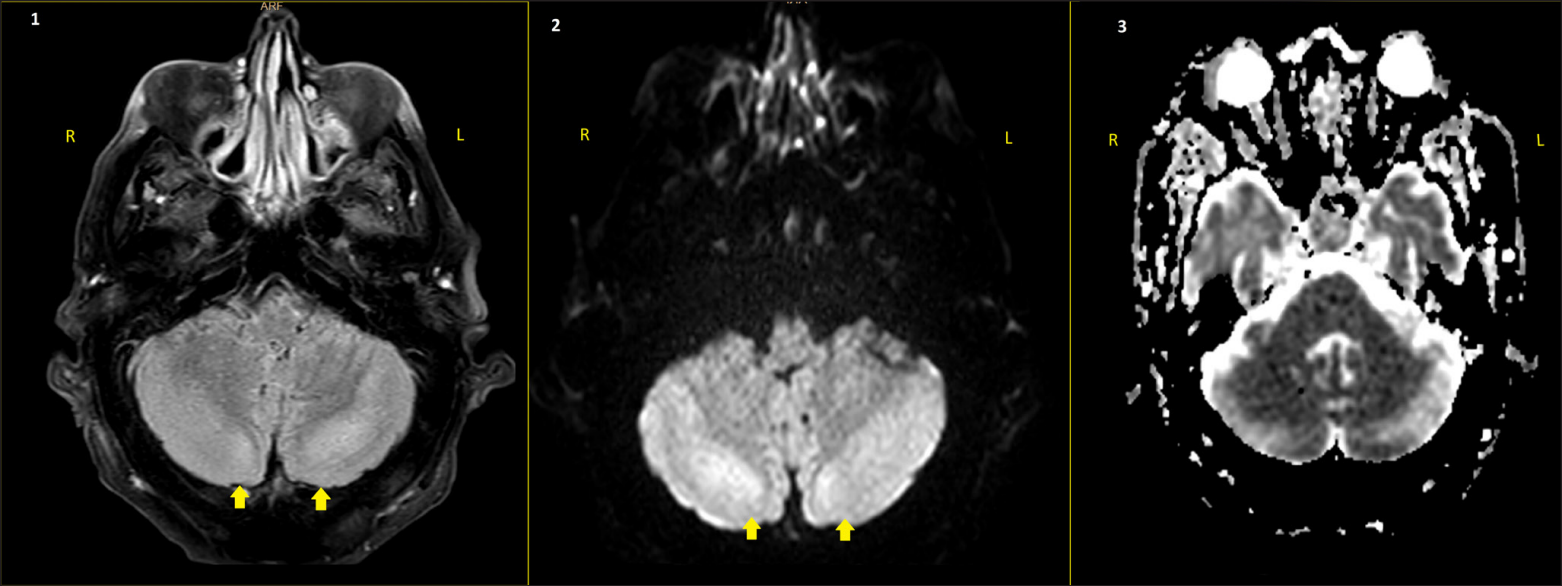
Brain MRI: 1. Hyperintensity is observed on T2-FLAIR sequences in the bilateral cerebellar hemispheres (yellow arrows), with marked asymmetric (left > right) distribution. 2–3. Hyperintensity is observed in DWI (2) and ADC (3) sequences, without clear restriction, which suggests of vasogenic edema at this level.
